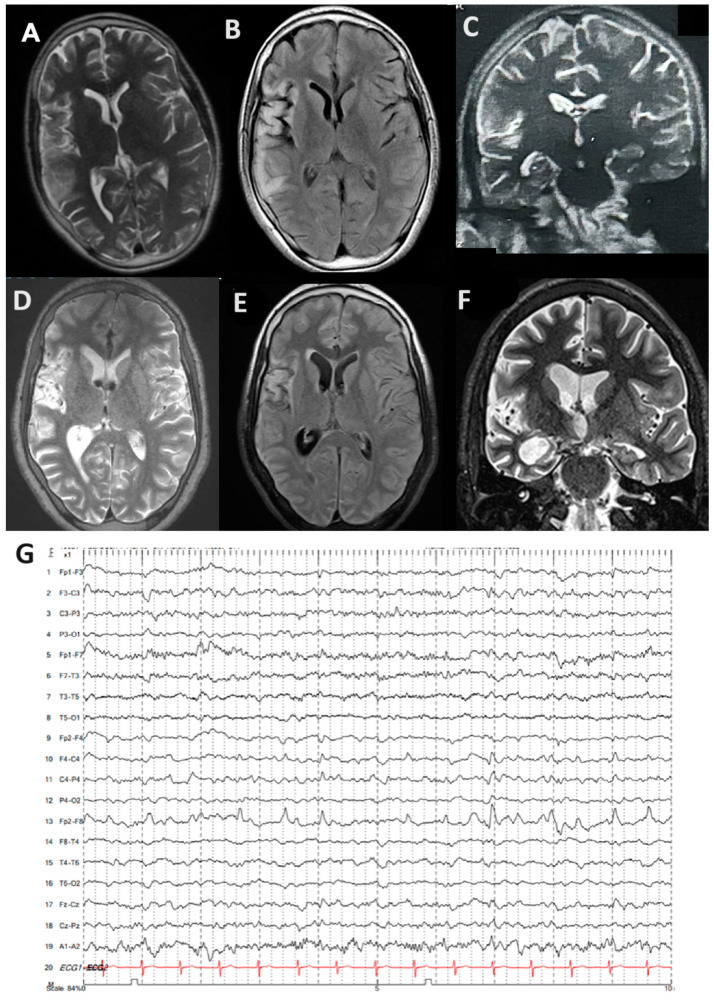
(A–G) MRI and EEG from patient with RE: (A–C) brain MR images highlighting T2 and T2 FLAIR. Hyperintensity in the right frontotemporal-insular region suggests subacute encephalitis with some degree of cortical atrophy; (D–F) brain MRI three months later shows extensive right hemisphere atrophy, predominating in the perisylvian region and affecting the caudate; (G) representative EEG with continuous rhythmic activity detected in the Fp2-F8 region.
